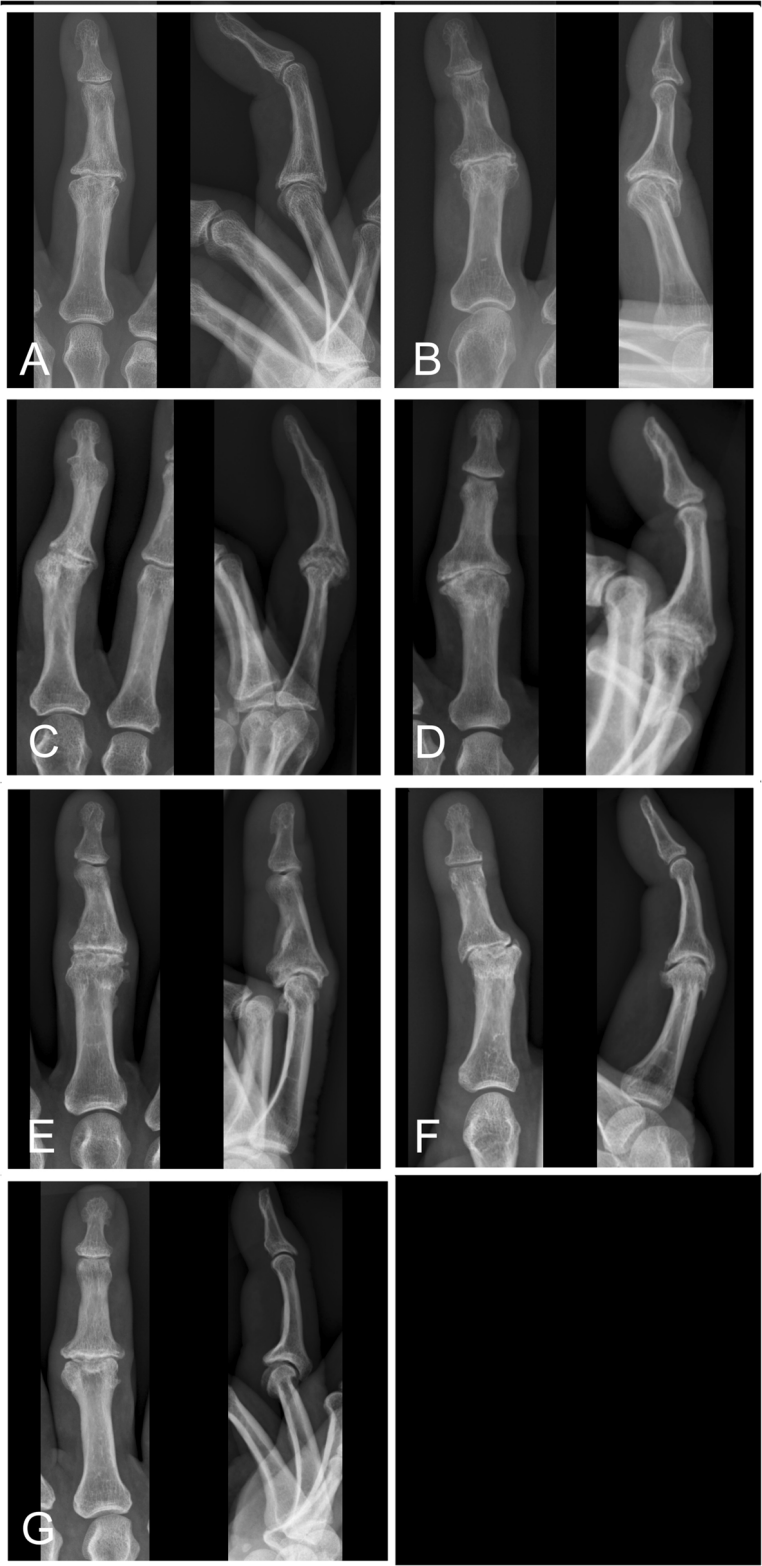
Radiographs of the reconstructed proximal interphalangeal joints in case 2–8 (a-g). No radiographs were obtained in case 1 before the patient passed away
